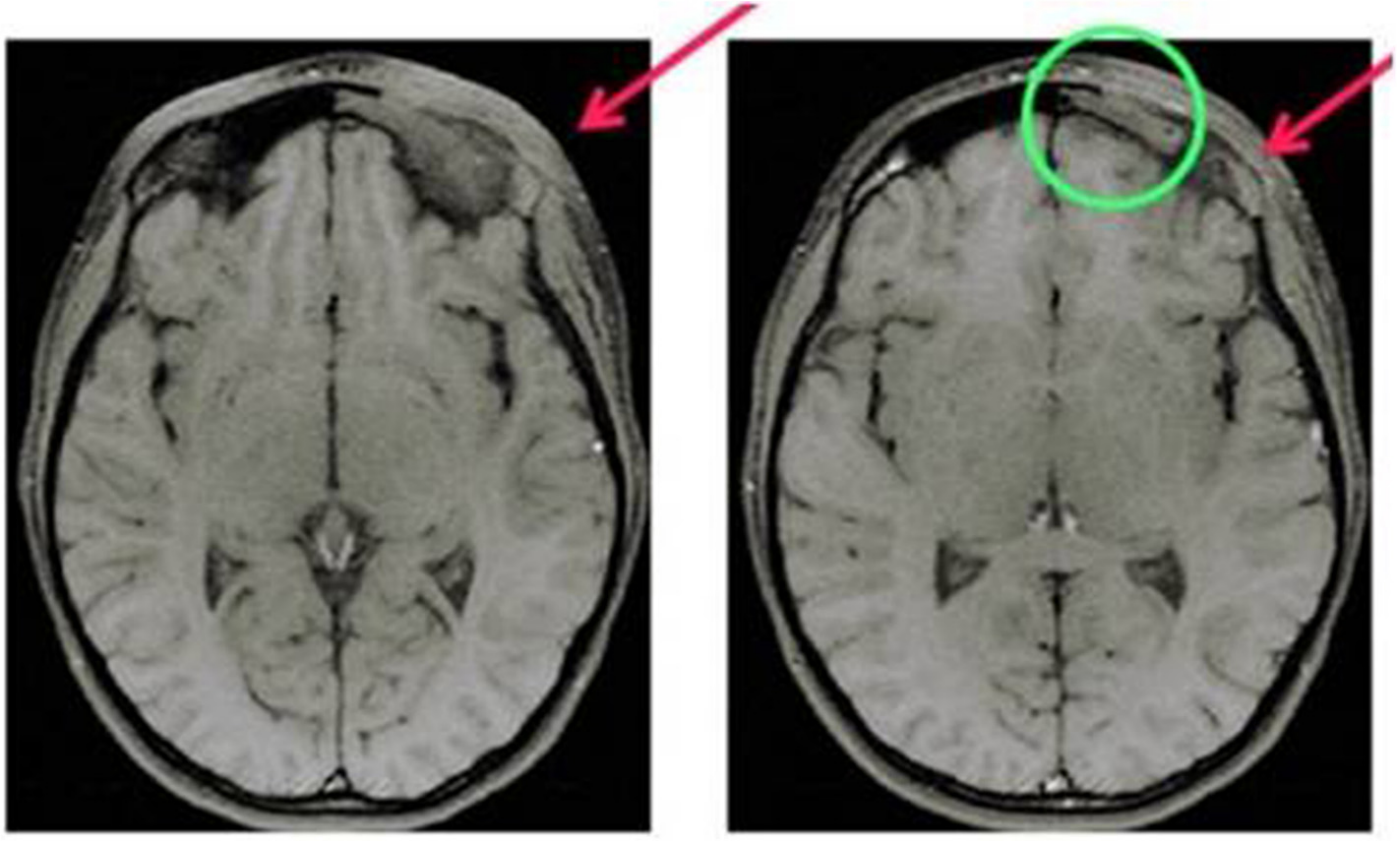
MRI: subacute inflammatory process at the level of the left orbital region with involvement of periorbital soft tissue and the bone structures
